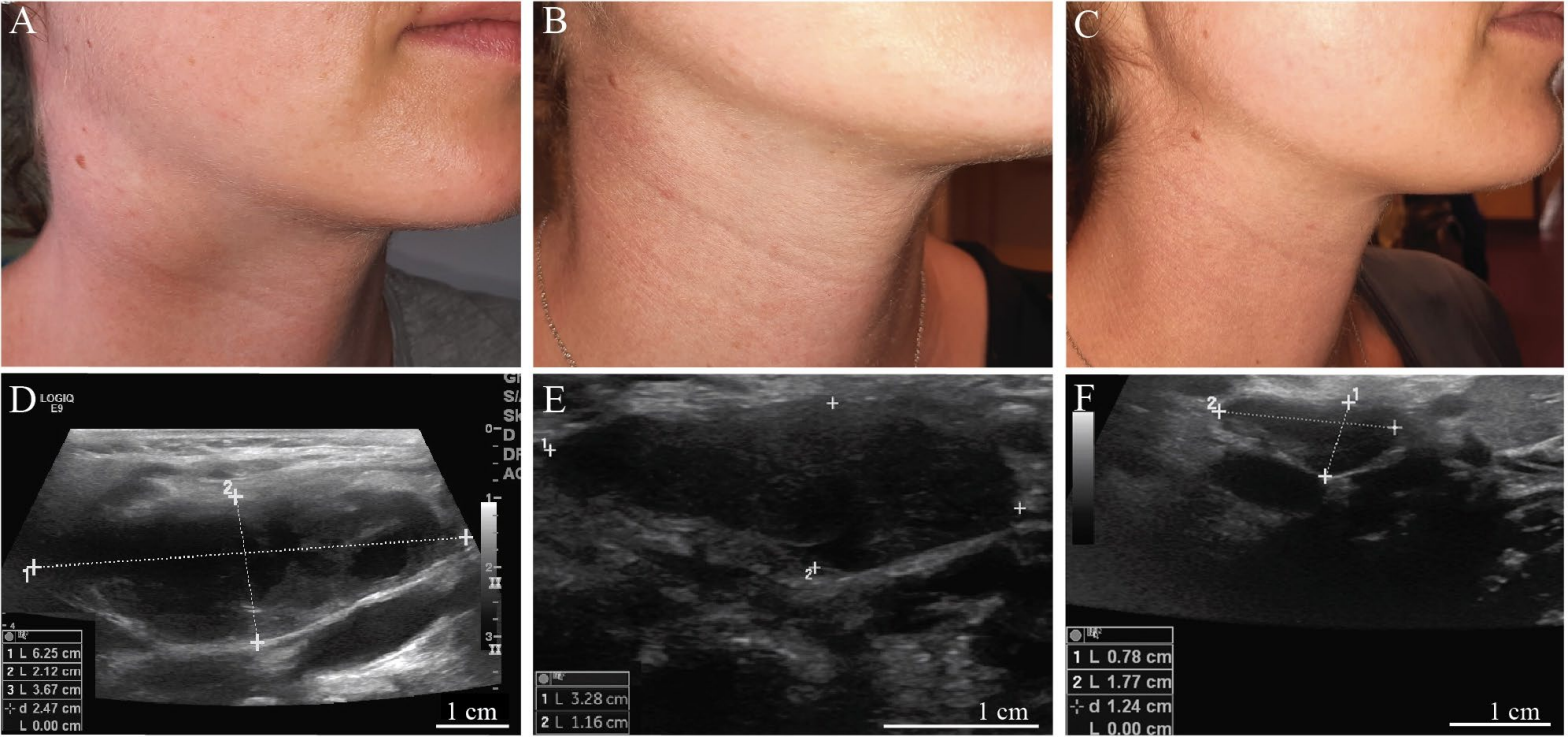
The clinical images as well as ultrasound controls at the day of admission (A, D), after 1 month of treatment (B, E) and after 6 months (C, F)
